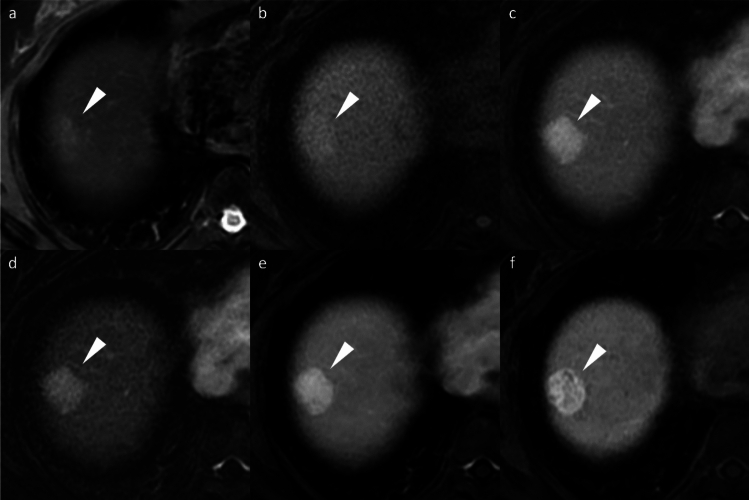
Focal nodular hyperplasia in a 47-year-old woman. A nodule (arrowheads) in segment 8 shows mild hyperintensity on the fat-suppressed T2-weighted image (a) and the pre-contrast image (b). The nodule displays non-rim arterial phase hyperenhancement (c) without portal venous washout (d), and prolonged hyperenhancement in the transitional phase (e). On the hepatobiliary phase image, the nodule appears distinctly hyperintense relative to the background liver (f). Typical imaging findings led to the diagnosis of focal nodular hyperplasia
